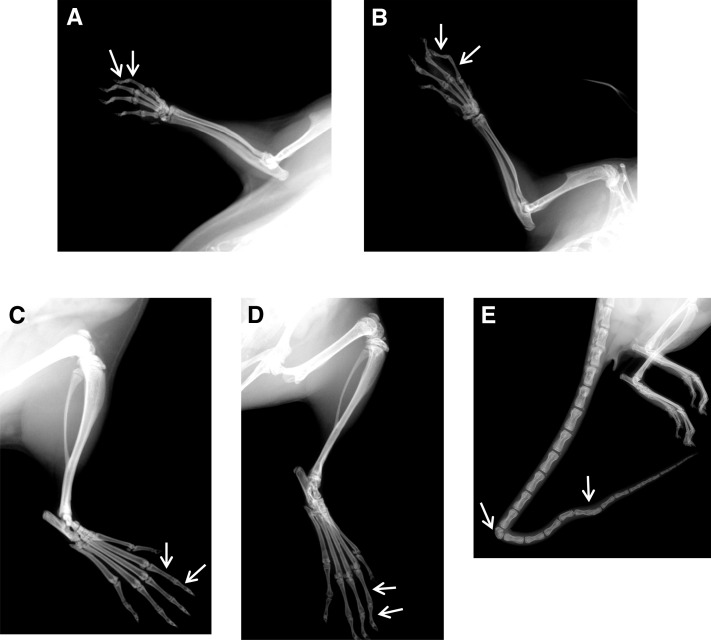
Arachnodactyly and kinked tail in skeletal morphology mutant 1 (*Skm1*). Radiographs of normal (A–C) and *Skm1* (B–D) manus (A, B) and pes (C, D). Phalanges are indicated by arrowheads. (E) Radiograph of the tail of *Skm1*. The hemivertebrae and fused vertebrae are indicated by arrowheads.
